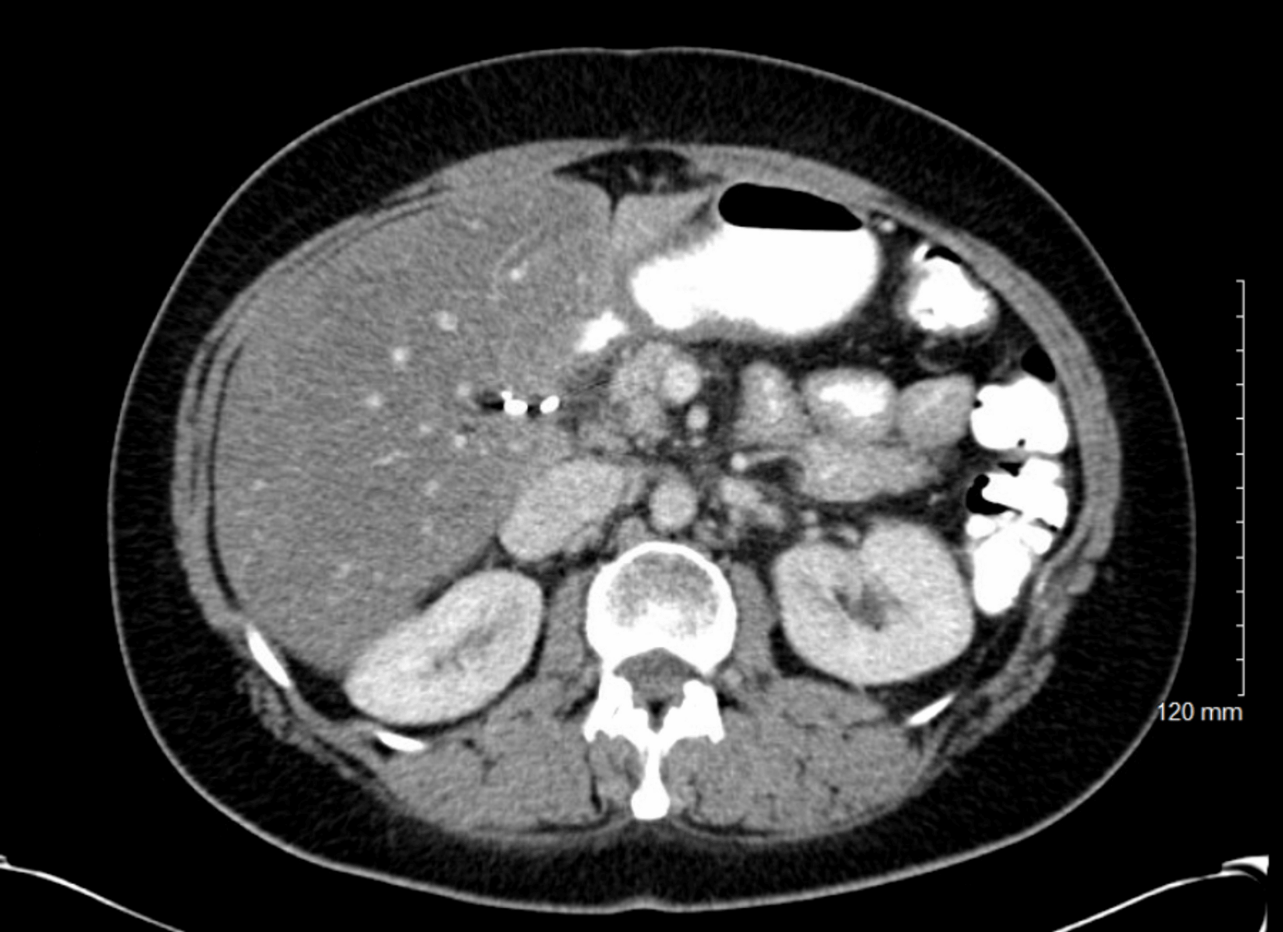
The CT showed complete response in the pancreas CT: computed tomography
